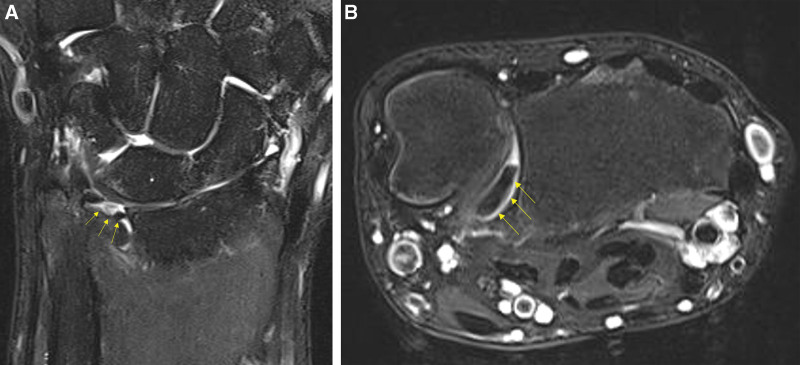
(A, B). T2-weighted magnetic resonance imaging (MRI) of the patient. In coronal view (A) it shows high signal intensity change and discontinuity in the triangular fibrocartilage complex (TFCC) central portion suggesting TFCC central and radial side tear (arrows). In axial view (B) it shows a torn TFCC flap is interposed in the distal radioulnar joint (DRUJ) at the volar side (arrows).
